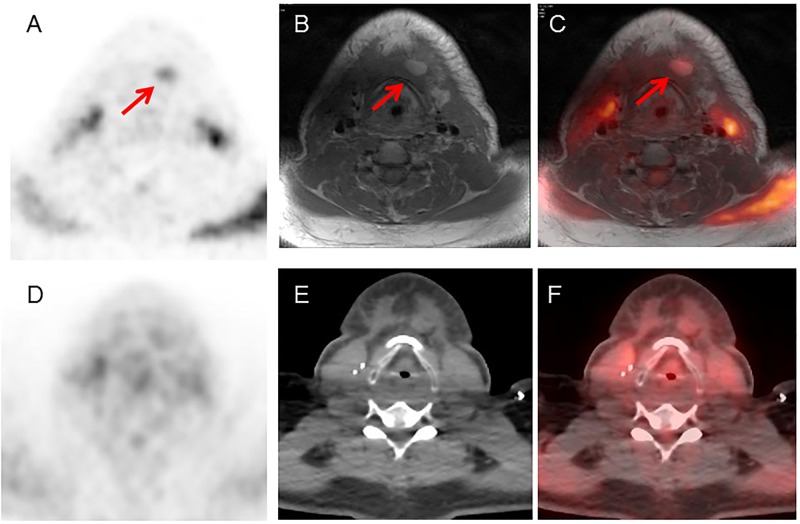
PET/MRI positive, PET/CT negative case. 32 year-old male with tongue cancer who underwent PET/CT for subsequent treatment strategy. (A) PET image from PET/MRI, (B) MRI image (T1-weighted image), (C) PET and MRI fused image, (D) PET image from PET/CT, (E) CT image, (F) PET and CT fused image. PET/MRI could detect the submandibular lymph node clearly, both because of better lesion to background contrast, but also due to superior MR contrast. PET/CT showed no abnormal uptake in this lesion, even though the node itself could be seen on PET/CT.
